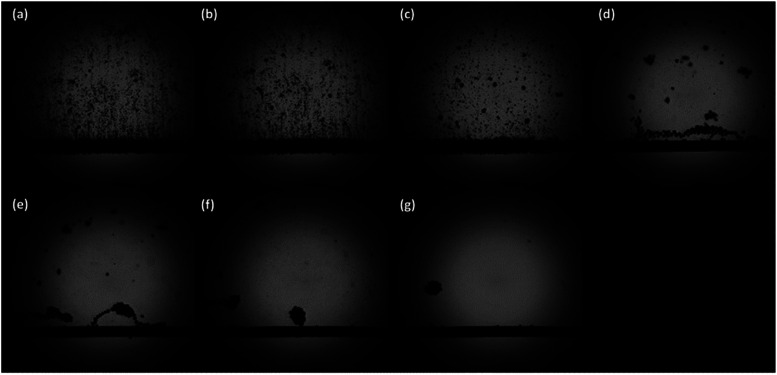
Hydrogen evolution on a Pt wire in the absence (top left corner) and presence of ultrasound (26 kHz, 100% ultrasonic amplitude). The applied potential was set at −1.30 V *vs.* RHE – (a) 0 μs, (b) 100 μs, (c) 200 μs, (d) 300 μs, (e) 400 μs, (f) 500 μs, (g) 600 μs. The time between each image is 10^−4^ s (100 μs) filmed at 10 000 frames per second. Reproduced with permission from ref. 1836. Copyright Elsevier 2020.
